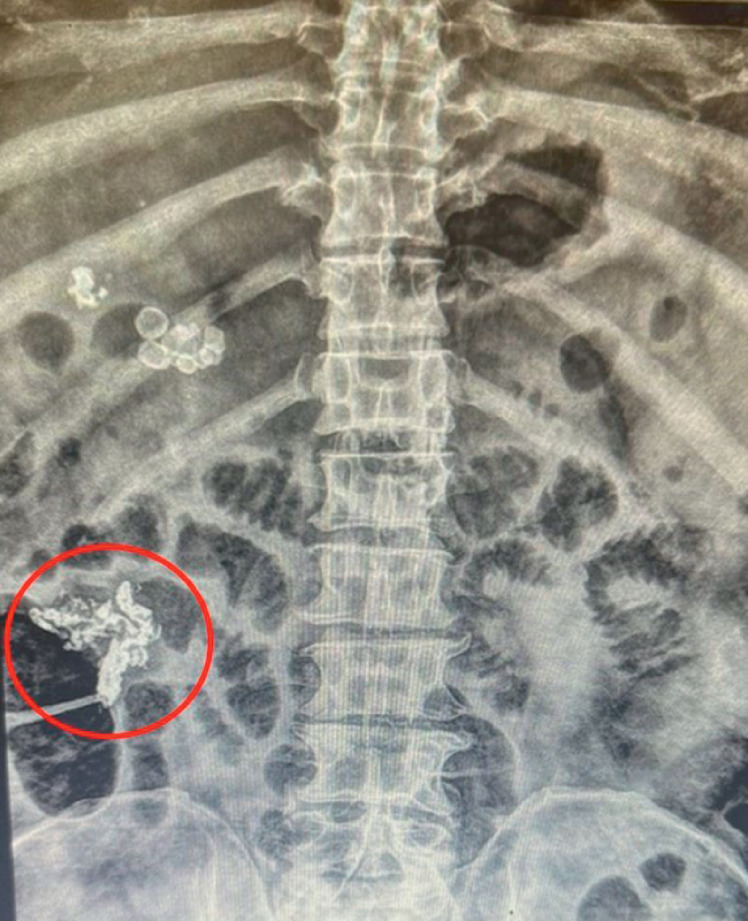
X-ray post-endoscopy confirms successful complete occlusion of the complex ascending colon varix and its contributors (red circle).
